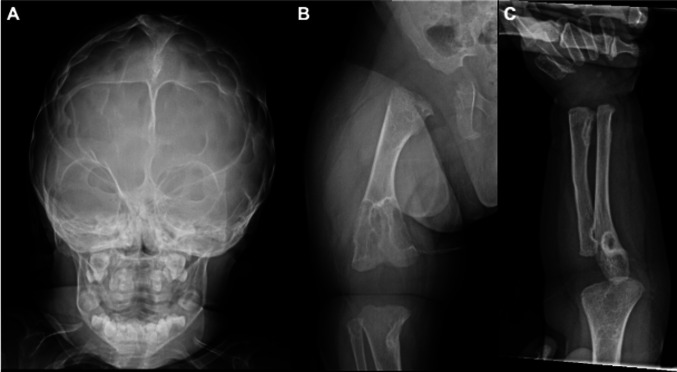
An 18-month-old with osteoglophonic dysplasia has radiographs demonstrating (A) “copper beaten skull” appearance from craniosynostosis, and nonossifying fibromas in the (B) distal femur, proximal tibia and fibula, and (C) the radius, ulna and humerus
